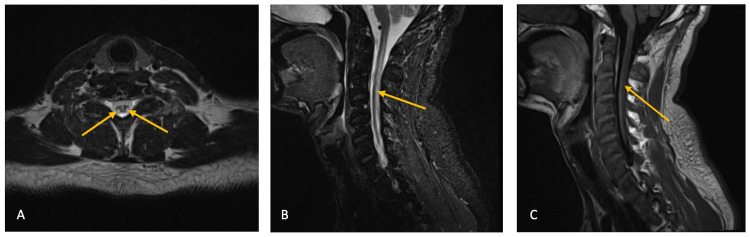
MRI of the cervical spine T2 sagittal (A) and T2 axial (B) MRI of the spine demonstrating symmetric bilateral high signal within the dorsal columns that is commonly described as the inverted "V" sign (arrows). T1 post-contrast image (C) confirmed that these were non-enhancing lesions (arrow) MRI: magnetic resonance imaging
